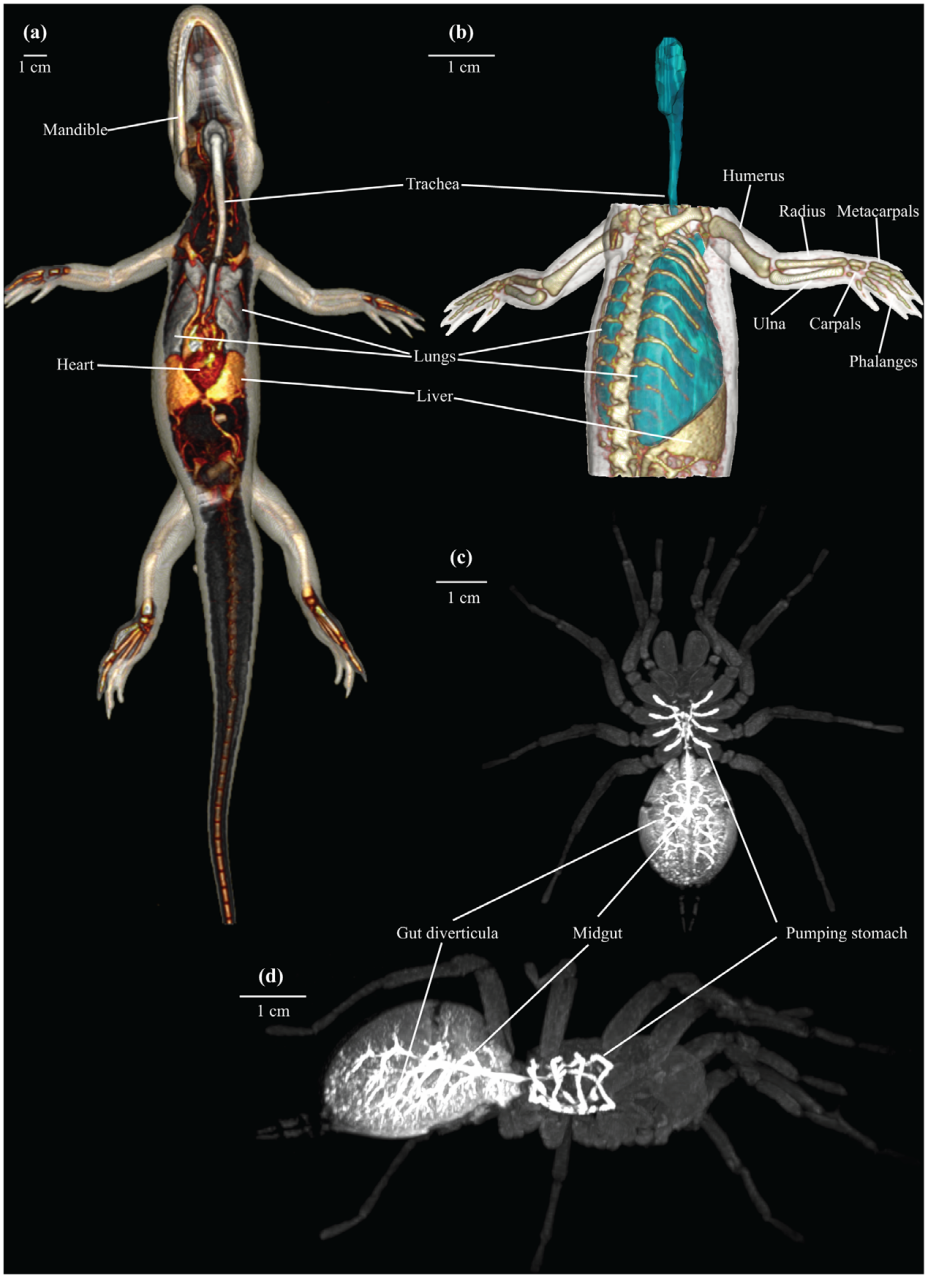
CT and MRI of organ structures. CT (a, b) and MRI (c, d) representations of organ structures. (a): Ventral view of American alligator (*Alligator mississippiensis*) with lungs (gray), liver (yellow) and heart (red) highlighted. (b): Ventrolateral view of American alligator (*Alligator mississippiensis*) with air-filled structures (lungs and trachea) highlighted blue. (c) and (d): Coronal (c) and lateral (d) view of whiteknee tarantula (*Acanthoscurria geniculate*) with the gastrointestinal tract enhanced following ingestion of MRI contrast agent.
